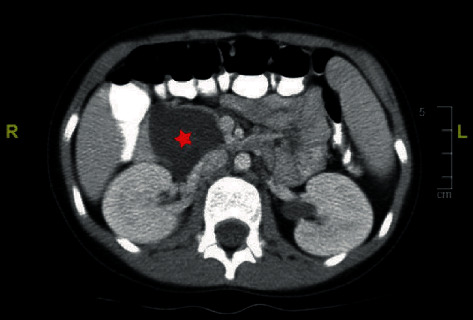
Computed tomographic scan of the abdomen revealed a large cystic lesion (star) in the region of pancreatic head.
